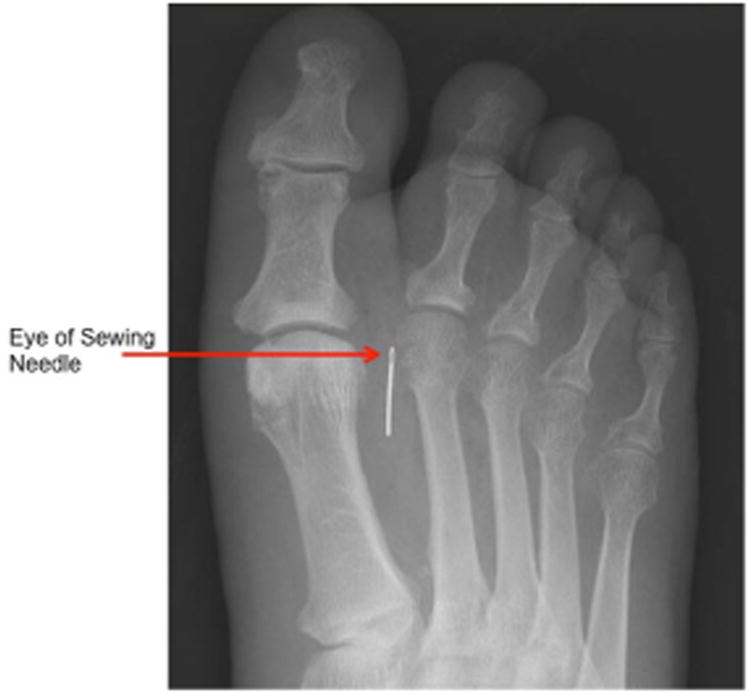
Foot X-ray shows a 3 cm linear metallic foreign body, a broken sewing needle in the soft tissues between distal first and second metatarsals.
